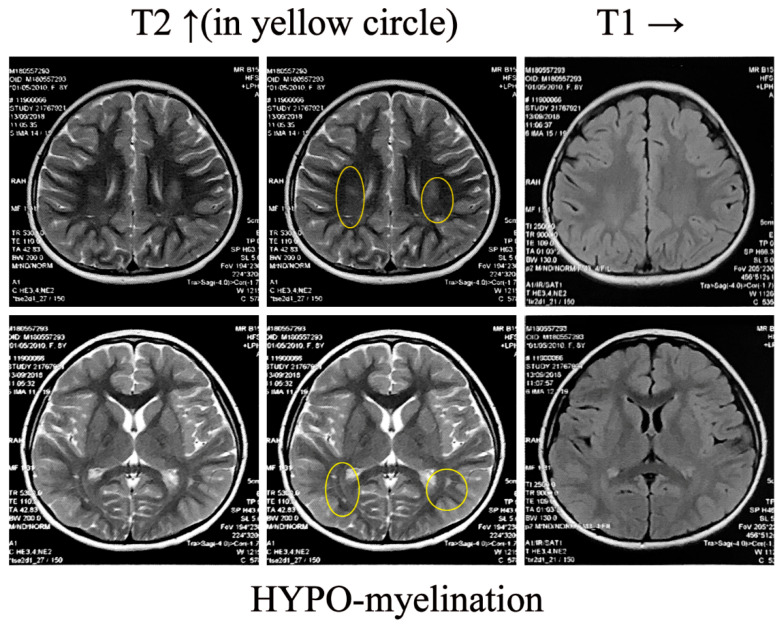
Leukoaraiosis with mild dysmyelination observed in bilateral parietal lobes. Brain MRI from the proband showed diffuse white matter hyperintensity on T2-weighted images (indicated by yellow circles), and the T1-weighted signal represented isointensity, which was consistent with a hypomyelinating leukodystrophy.
